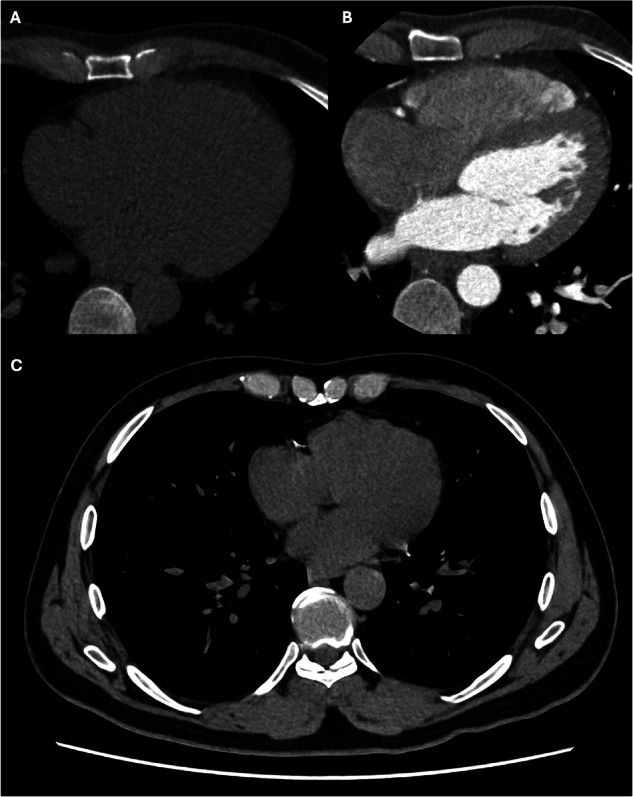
Development of CAD in a 43-year-old male smoker, with atypical chest pain, hypercholesterolemia, and a family history of CAD. At baseline, the coronary artery calcium score was zero (A), and the coronary CT angiogram was normal (B). Non-contrast CT chest (C) 10 years later showed calcified plaques in the right coronary artery and left circumflex coronary artery
